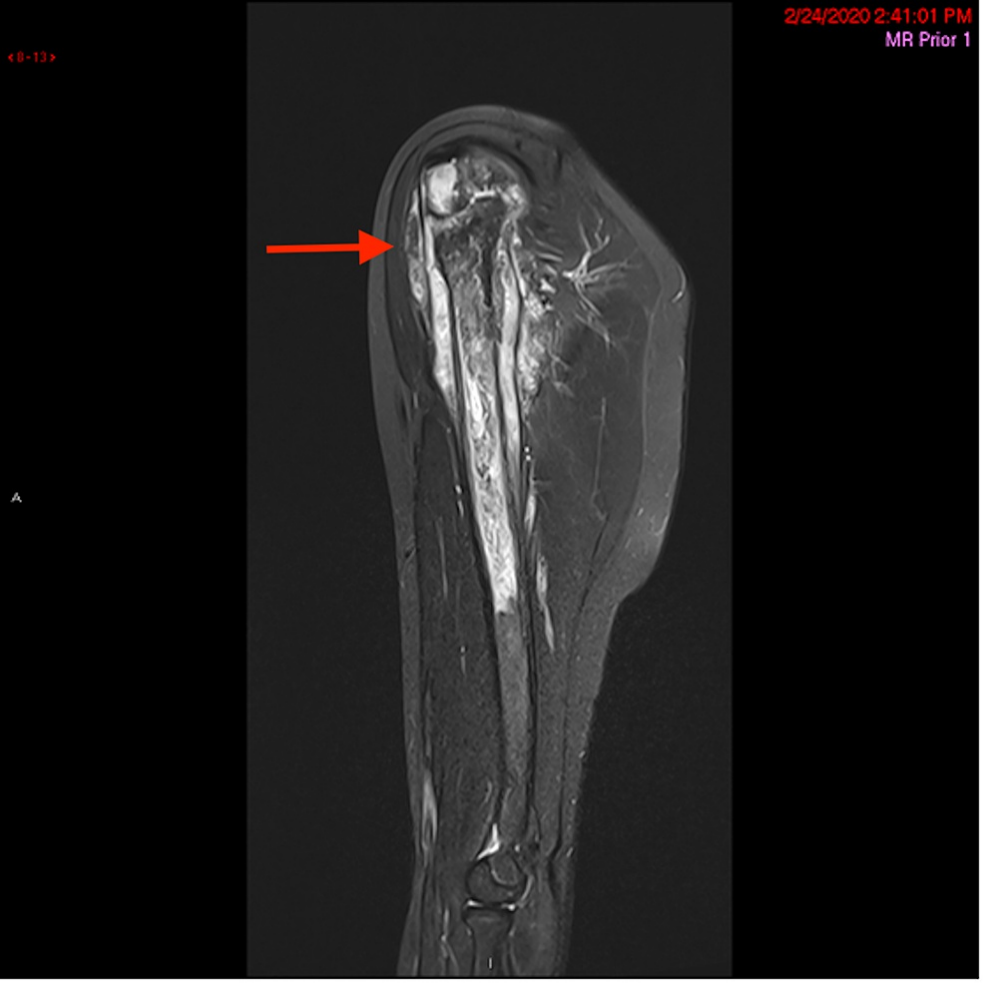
MRI of the right upper extremity. Sagittal fluid sensitive sequence with fat saturation shows increased T2 signal involving the epiphysis, metaphysis, and diaphysis of the proximal humerus. Note the subperiosteal soft tissue extension and adjacent muscle edema (red arrow).
